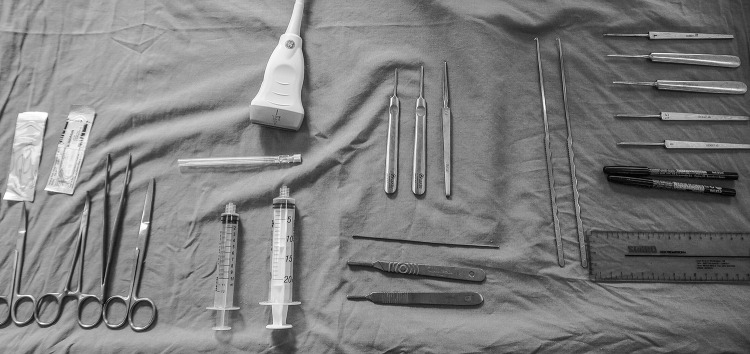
Instruments for the UGAFDS approach; ultrasound device; dissection material; 20 gauge needles, syringe; V-shape; hook knife
